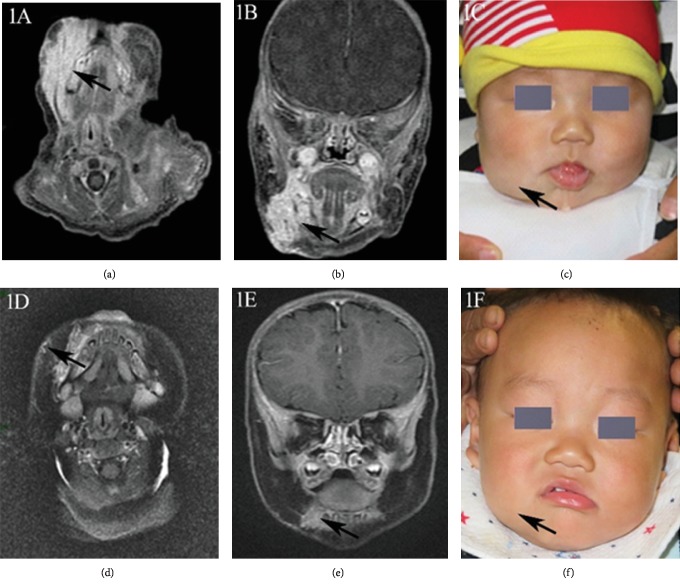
An 11-week-old boy with a deep IH on the right maxillofacial region. (a–c) Before propranolol treatment, (a–b) axial and coronal MRI T_1_WI enhancement showed obvious high signal of the right maxillofacial hemangioma. (d–f) After 8 months of propranolol treatment, (d, e) axial and coronal MRI T_1_WI enhancement showed only a few residual lesions.
